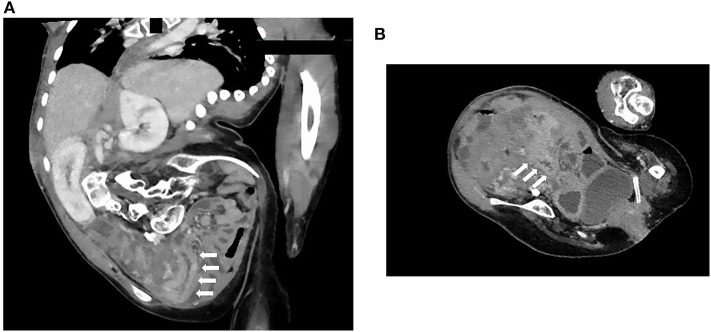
Abdominal contrast medium-enhanced computed tomography. (A,B) The images revealed an effect of enhancing the mucosal surface and the edematous wall thickening localized from the terminal ileum to the cecum. White arrows indicate the terminal ileum and cecum.
